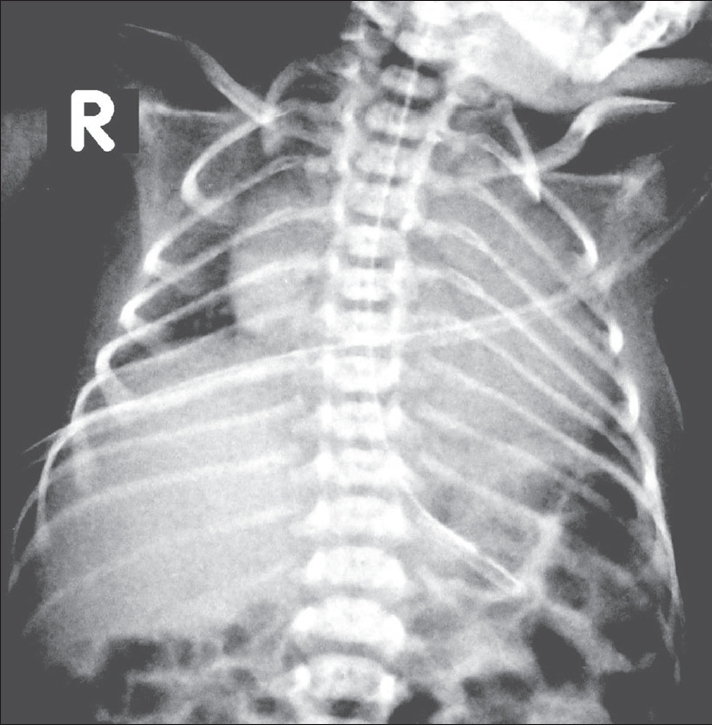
Chest radiograph on day 1
